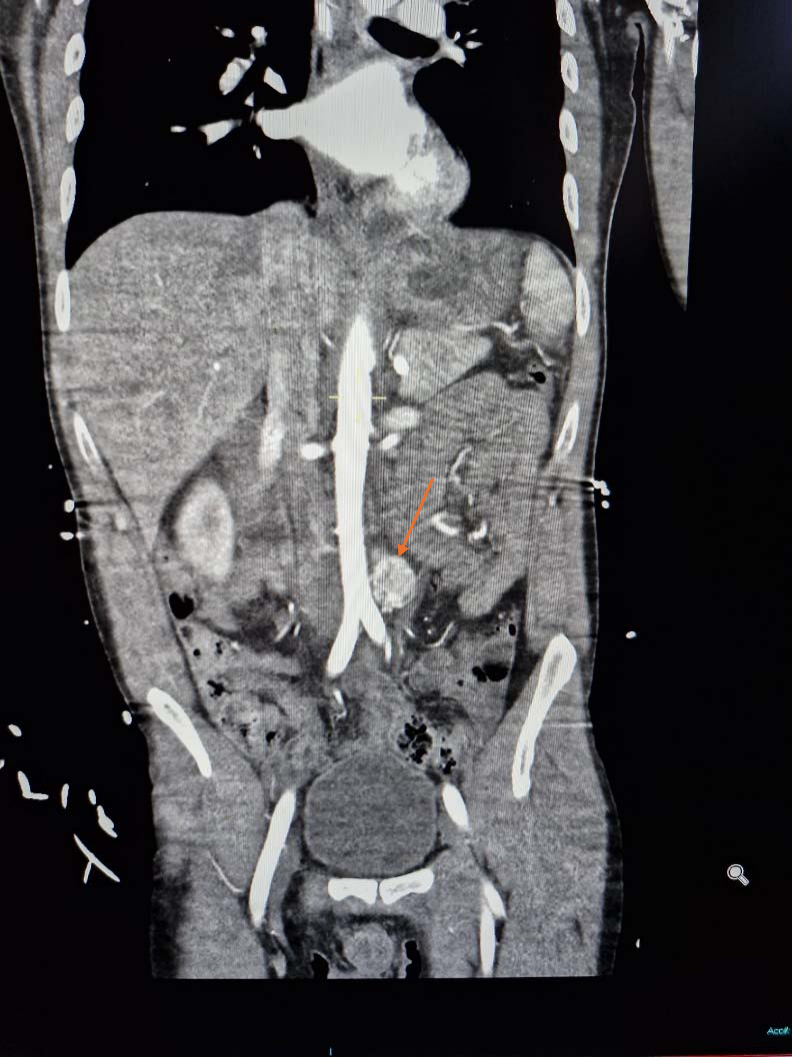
Contrasted CT of the abdomen and pelvis in arterial phase, coronal plane, revealed a similar mass, contrast enhanced heterogenous lesion with well demarcated borders, measuring ⁓2 × 2 × 3 cm, situated at the left para-aortic region.
